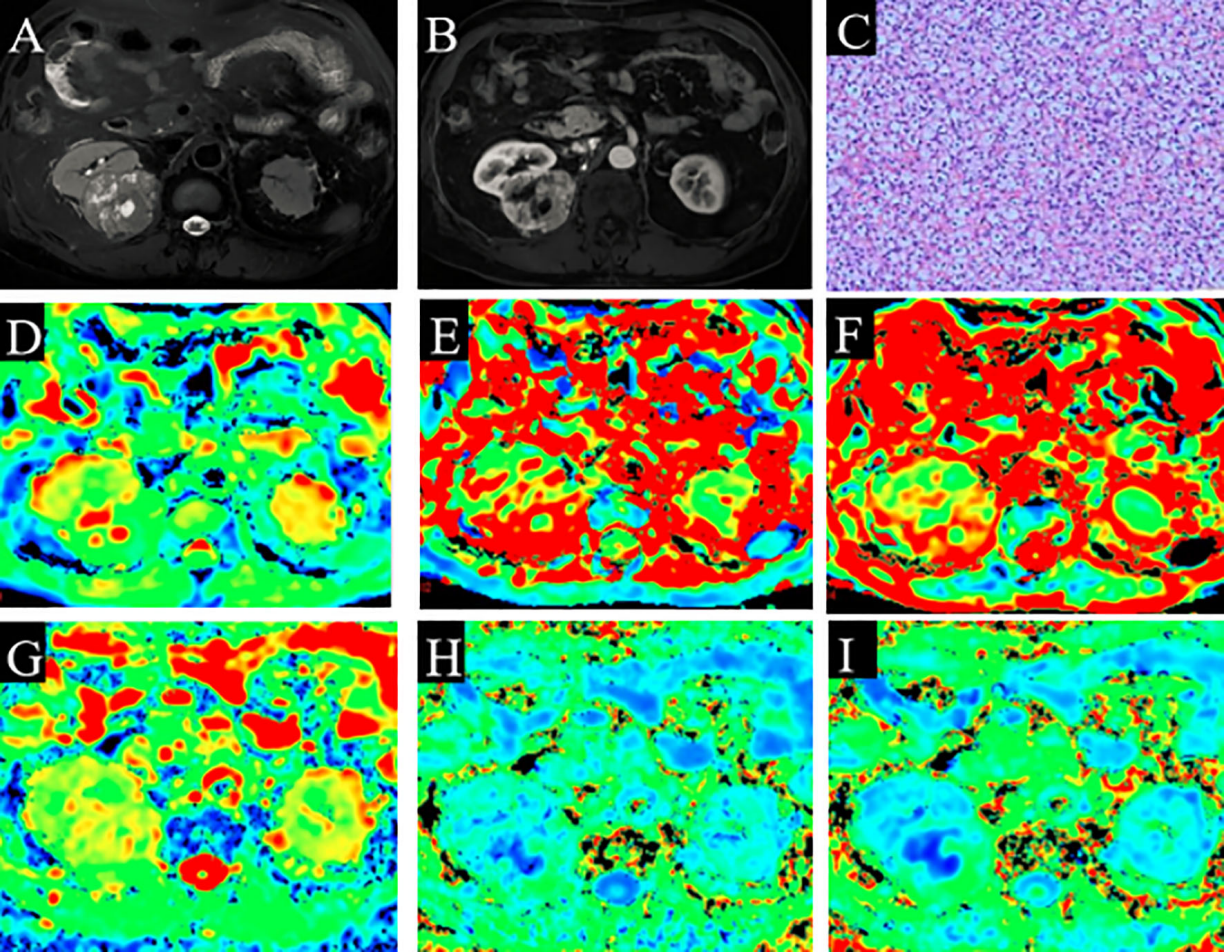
A 60-year-old man with ccRCC (grade 1 and stage 1). (A) Slightly high signal intensity on fat-saturated T2WI. (B) The lesion shows enhancement on solid part of ccRCC. (C) Pathological analysis confirmed ccRCC (grade 1). (D) D map. (E) D* map. (F) f map. (G) MD map. (H) KA map. (I) MK map. The necrotic areas in the center of the ccRCC. ccRCC, clear cell renal cell carcinoma; T2WI, T2-weighted imaging; MD, mean diffusivity; KA, kurtosis anisotropy; MK, mean kurtosis.
